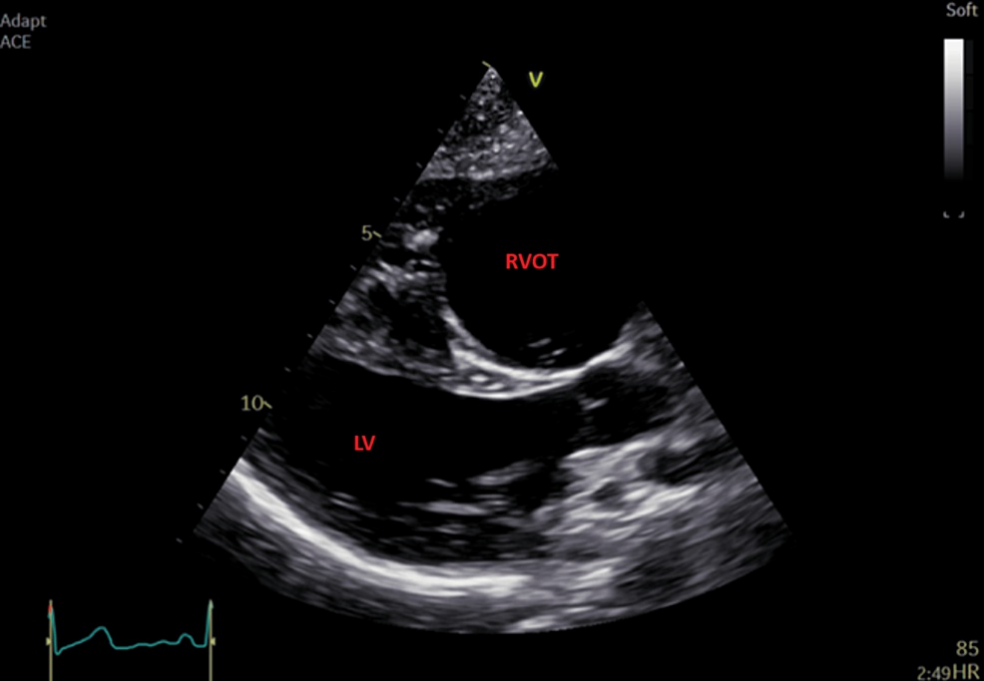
Parasternal long-axis view on TTE showing dilated RVOT. RVOT: right ventricular outflow tract; LV: left ventricle; TTE: transthoracic echocardiogram
